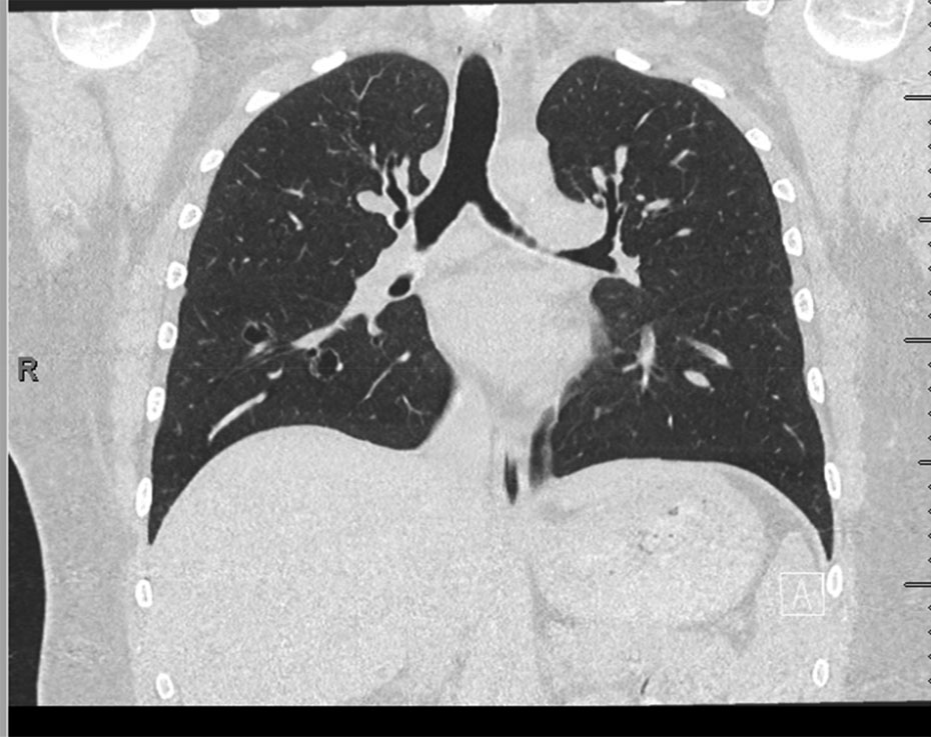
The figure shows a computed tomography scan of the patient's following 9 weeks of IV bevacizumab. The 14 mm right lower lobe nodule had resolved.
